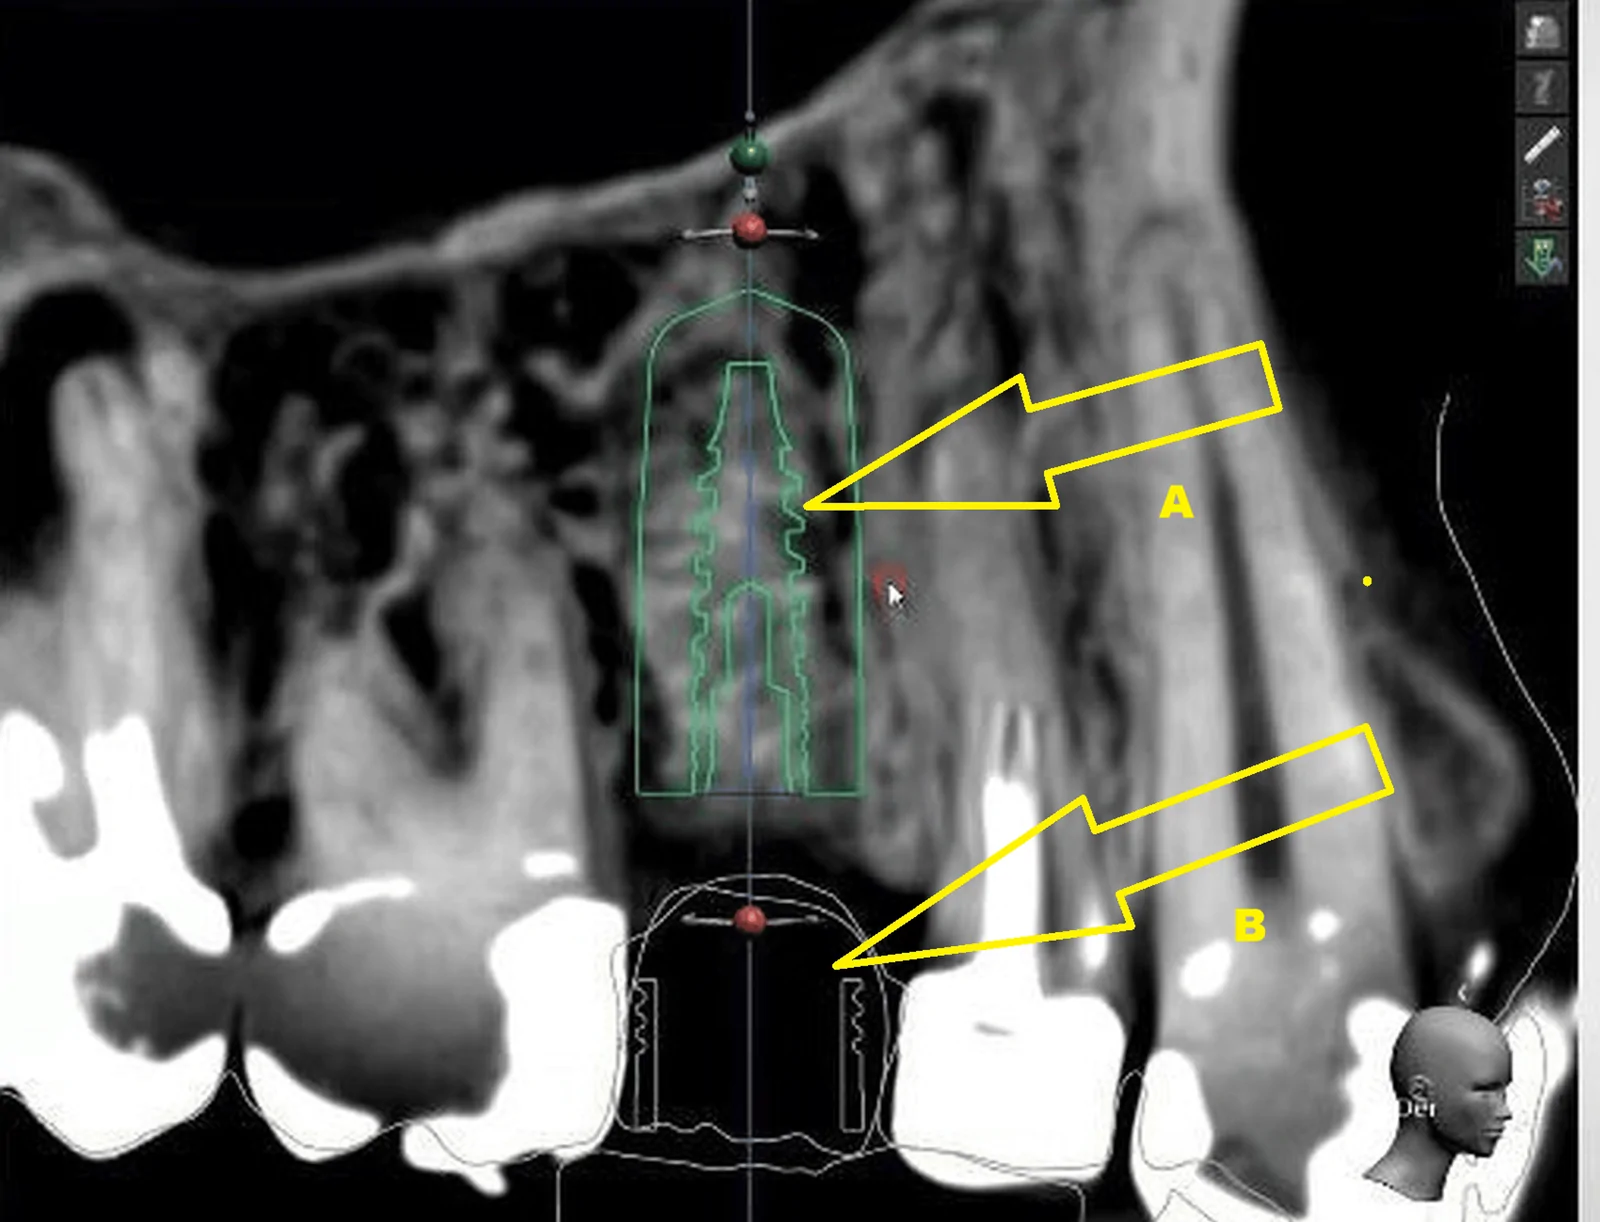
Continued CBCT-guided prosthetically driven implant planning for the maxillary right second premolar site. CBCT-based treatment planning was continued to evaluate the proposed implant position and future prosthetic restoration in this medically complex ASA III patient. Arrow A indicates the virtually planned implant, including its angulation, depth, and relationship to the surrounding anatomical structures. Arrow B indicates the digitally planned crown, which was used to guide prosthetically driven implant positioning and optimize the final restorative outcome. CBCT: cone-beam computed tomography; ASA: American Society of Anesthesiologists
